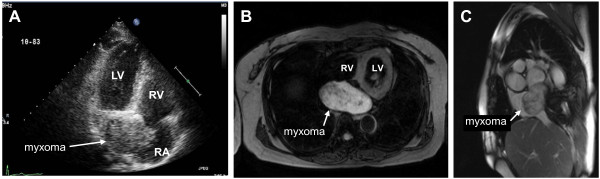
The large tumor, occupying both atria, was shown in the transthoracic echocardiography (A) and the magnetic resonance scan (B,C).
